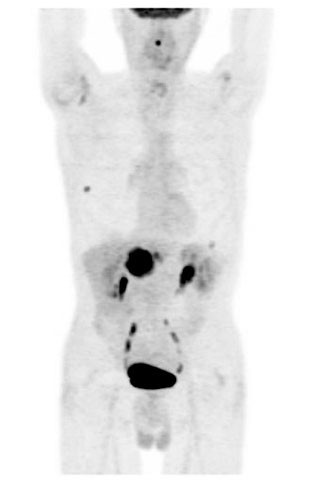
FDG PET-CT maximum intensity projection image showing three FDG-avid pulmonary nodules.
